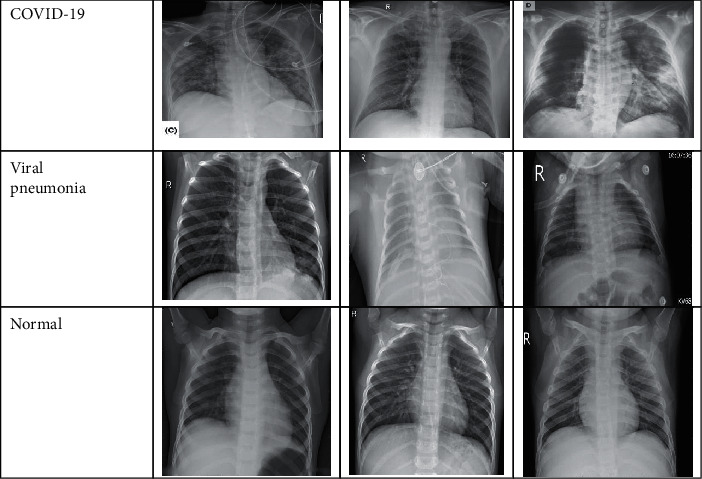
X-ray samples of COVID-19 data set.
